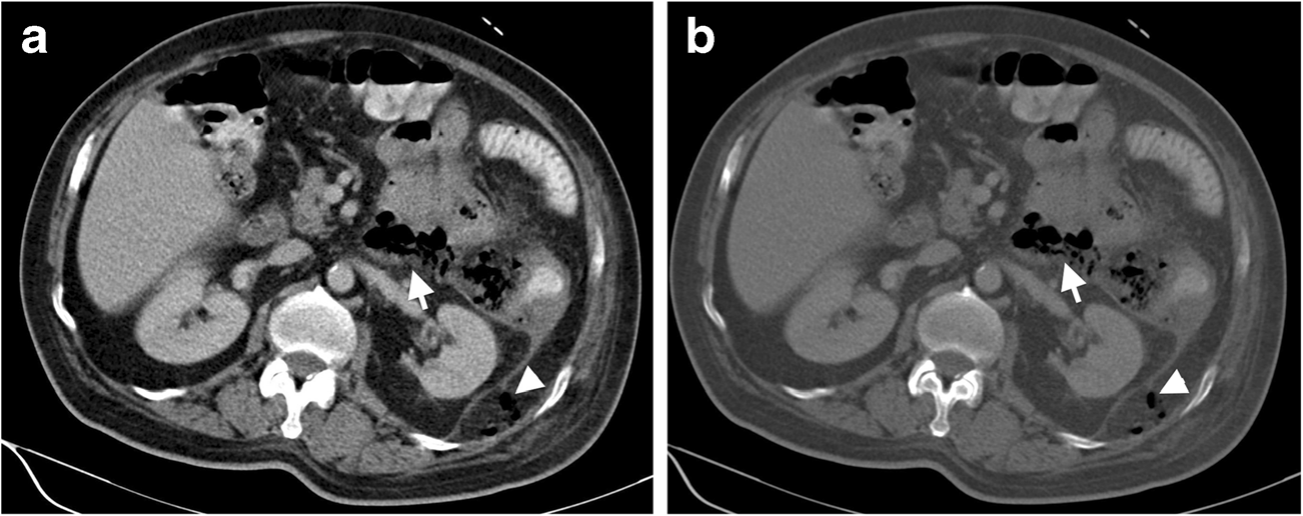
Seventy-two-year-old female with free perforation complicating diverticulitis. Axial contrast enhanced CT images at parenchymal window (a) and bone window (b) demonstrate a ‘dirty mass’ (*arrows*) formed by stool nearby sigmoid colon diverticulitis. Free air (*arrowheads*) and inflammatory fat stranding resulting from perforation of diverticulitis are seen posterior to the pararenal fascia
